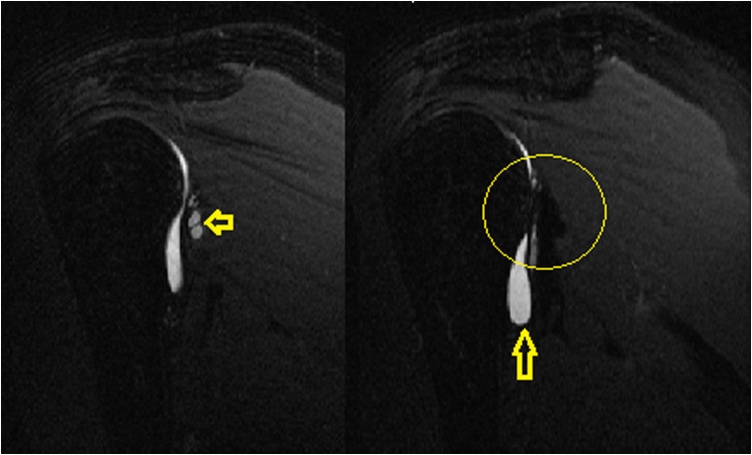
Sagittal MRI cuts of the right shoulder showing posterior cyst formation with the posterior labral tear of the glenoid.
